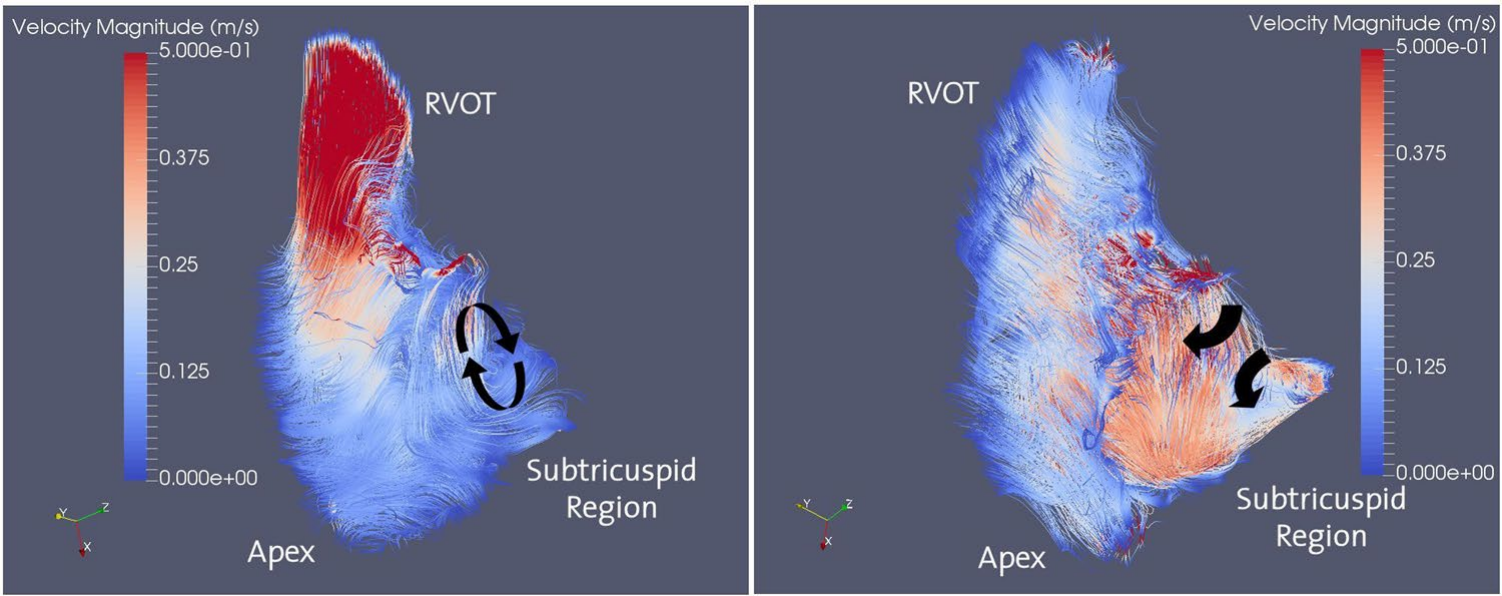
Streamlines color coded with velocity magnitude along the RV at peak systolic phase (left) and mid diastolic phase (right) obtained using *in vivo* PC-MRI in a healthy athletic male proband at resting conditions. Red color corresponds to high velocity and the blue color represents slow velocity regions. The PC-MRI data of the healthy male volunteer is used for qualitative representation of the blood flow and as a reference for validating the *in vitro* flow patterns of the silicone model (see Methods section). Recirculation regions at ventricular filling are marked with arrows.
